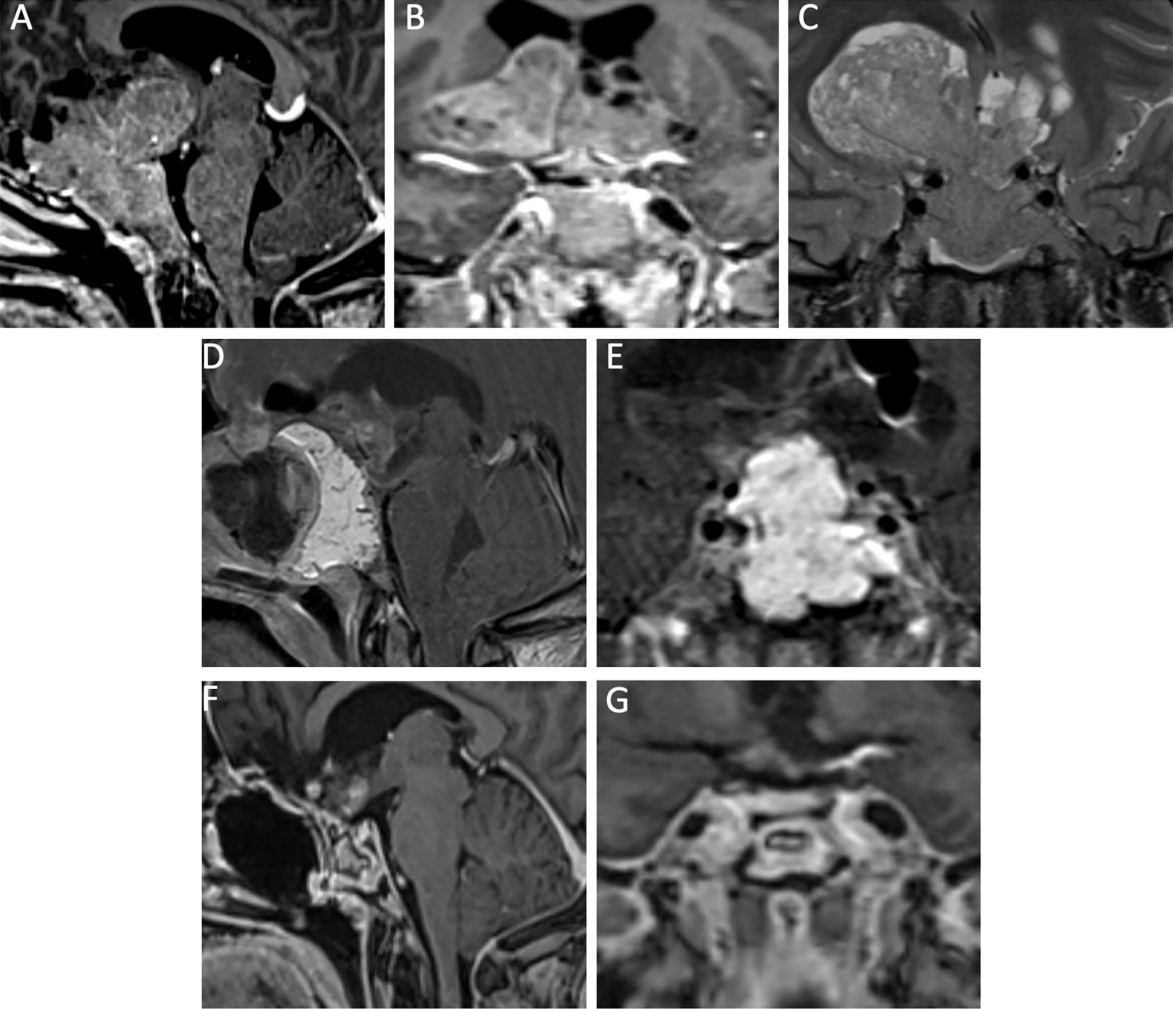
A 43-year-old woman with a giant GH-secreting pituitary adenoma. Preoperative MRI, T1-post gadolinium contrast, featuring sagittal (A) and coronal (B) reveal a massive, invasive tumor, invading into the brain parenchyma, bilateral cavernous sinuses, and clivus. The T2 coronal sequence (C) shows the invasion into the right frontal lobe. Immediate postoperative MRI, T1-post gadolinium contrast, sagittal (D) and coronal (E) views shows radical resection of tumor, with scant residual in the cavernous sinus. A fat graft and nasoseptal flap were employed. 1-year postoperative there is a small amount of residual tumor in the cavernous sinuses, as demonstrated by the sagittal (F) and coronal (G) MRI T1-post gadolinium contrast. The patient achieved biochemical remission after initiation of octreotide. No radiation was needed.
